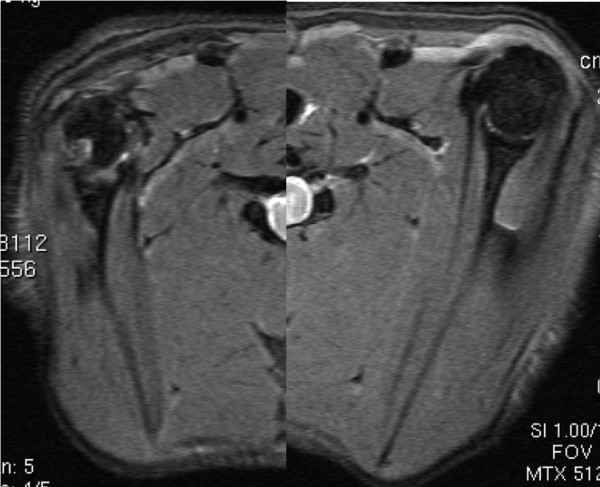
Shoulder MRI 16 weeks after surgery showing a loss of relationship between the glenoid -which presents a normal version- and a marked deformed humeral head. An advanced right glenohumeral joint hypoplasia is present. The distinctive shoulder dysplasia found in humans is absent.
